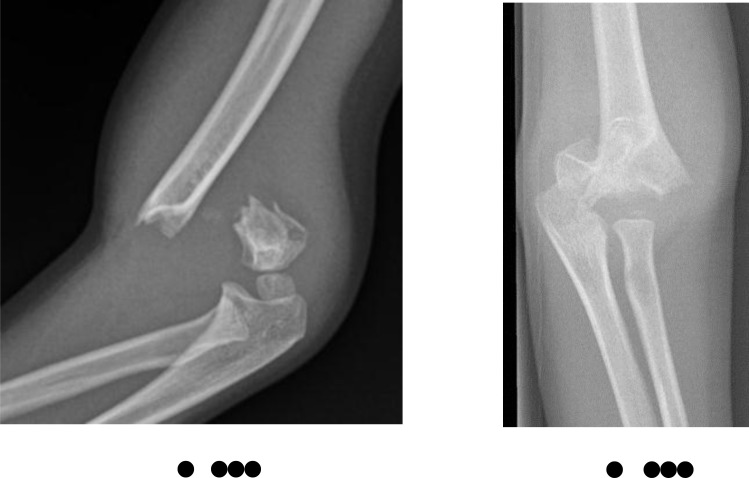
AP and lateral radiographs of Wilkin’s-modified Gartland Grade 3 supracondylar humeral fracture.
